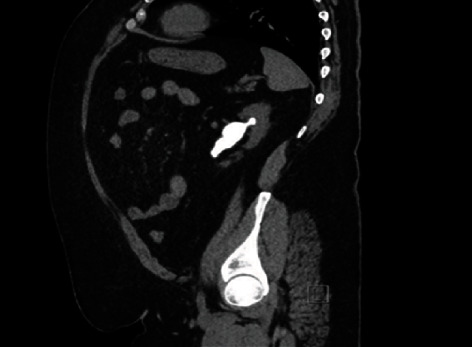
CT KUB demonstrating left staghorn kidney stone.
